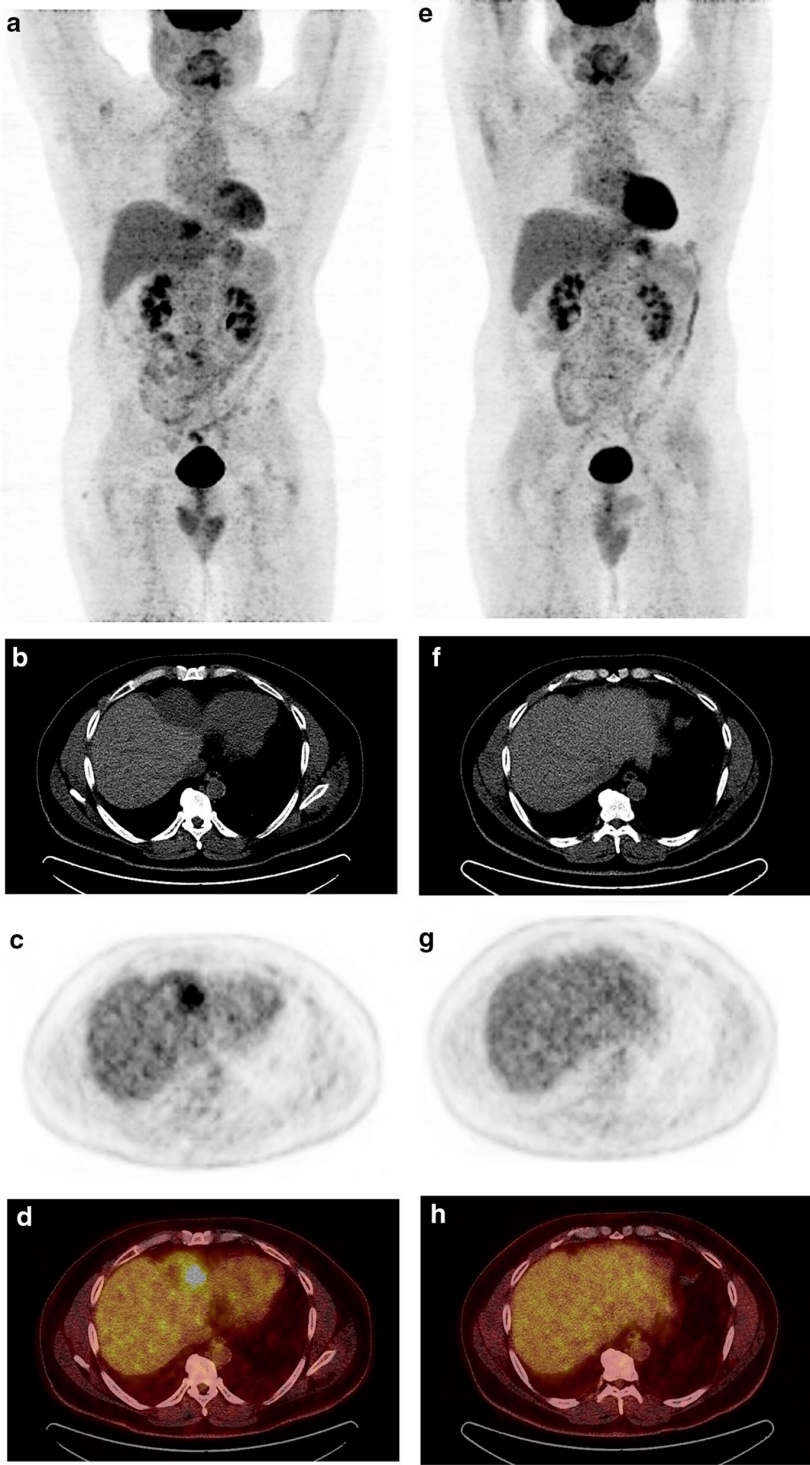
PET/CT imaging prior to (a–d) and after 10 weeks (e–h) of sunitinib therapy. Prior to treatment, whole body PET (a) revealed a large hypermetabolic lesion in the dome of the liver, a PET-avid sub-cm L hepatic lesion, and hypermetabolic activity in the abdominal wall near the site of previous surgical resection. On cross sectional CT imaging (b) the large hepatic lesion measured 5.9 × 5.1 cm in maximal dimension, and the corresponding area on PET imaging (c) and fused PET/CT (d) revealed an SUV_max_ of 8.4. Both liver lesions were no longer detectable on repeat imaging (e–h), and the surgical site hypermetabolic activity decreased (e), consistent with resolving post-operative changes
